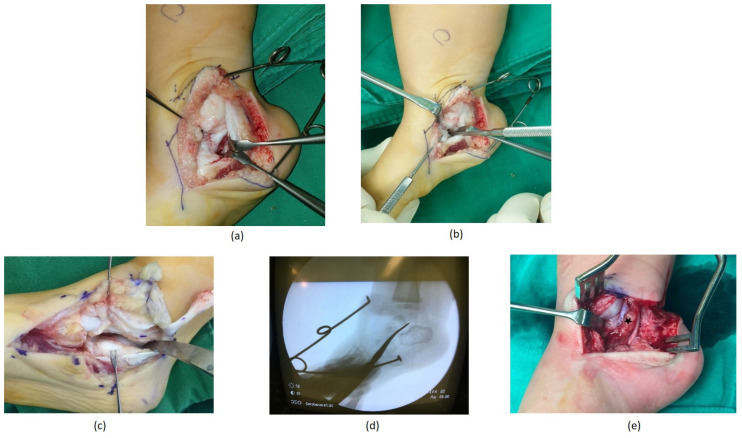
Intraoperative images showing the medial side of the foot. (a) Through the medial incision, the flexor digitorum longus tendon retracts with the periosteum to expose the medial aspect of the talocalcaneal joint; (b) we attempted to define the extent of the coalition using a blunt instrument; (c) after excising the coalition with a scalpel, the entire subtalar joint is visible with normal joint cartilage and the range of subtalar joint motion improves drastically; (d) intraoperative fluoroscopic radiographic confirmation of the entire coalition excision; (e) to prevent coalition recurrence, a fibrin sealant was used as the interposition material (marked with an asterisk).
